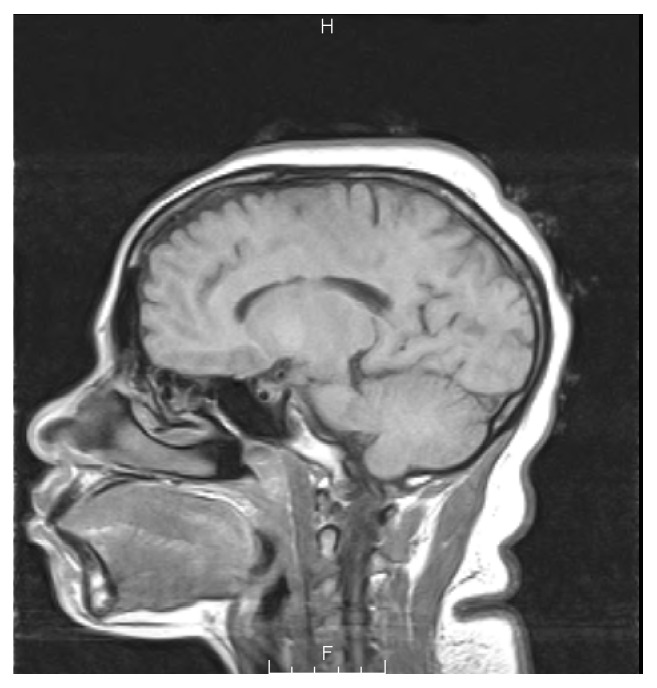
Sagittal T1 diffusion-weighted MRI of the pituitary gland.
